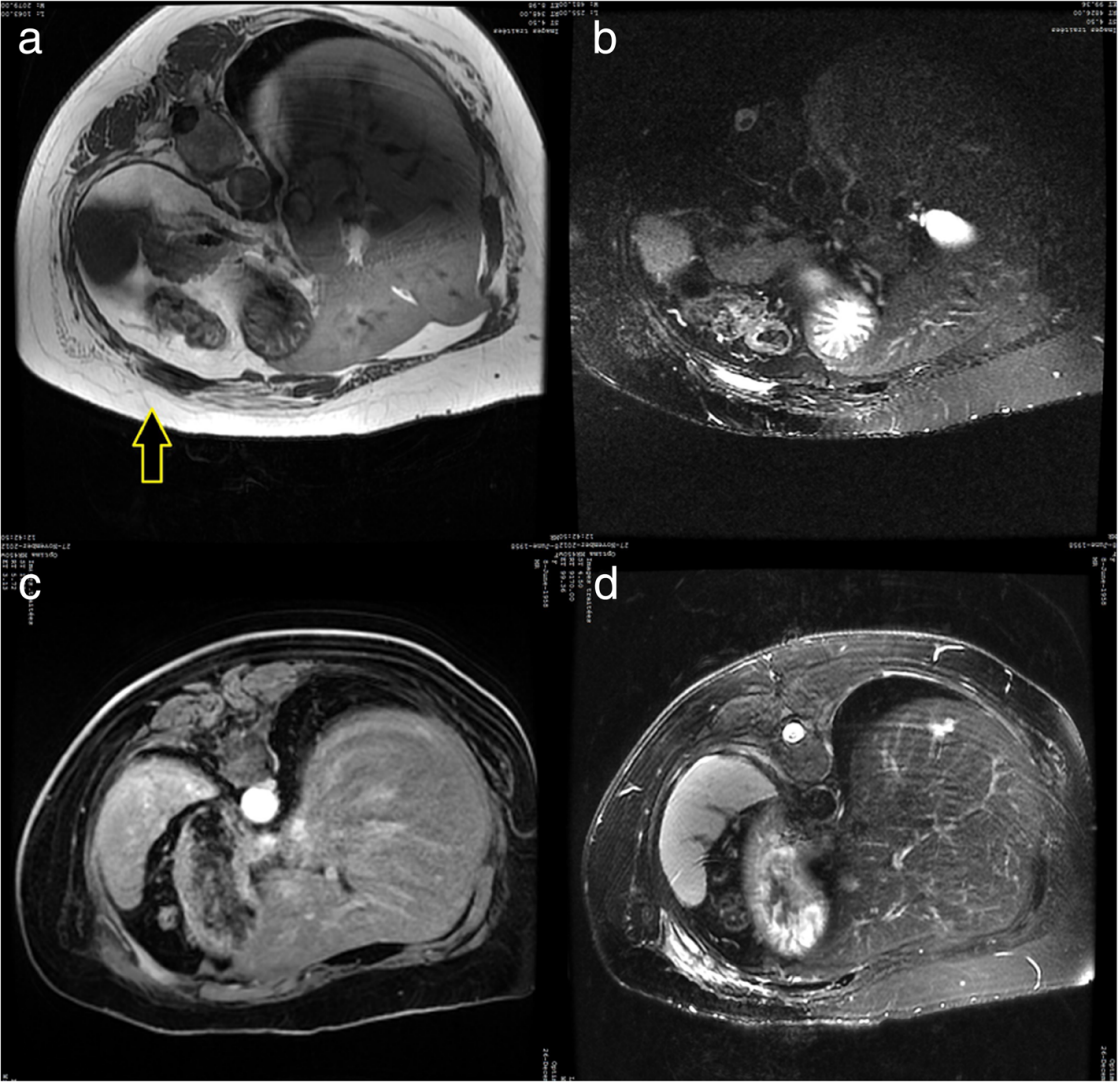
Patient 3. a, b Axial T1-weighted and T2-weighted fat-suppressed MR images showing a bone metastasis of the 7th rib before the procedure (prone position); c, d. Axial T1 with contrast MR images and T2-weighted fat-suppressed showing the lesion after the procedure
